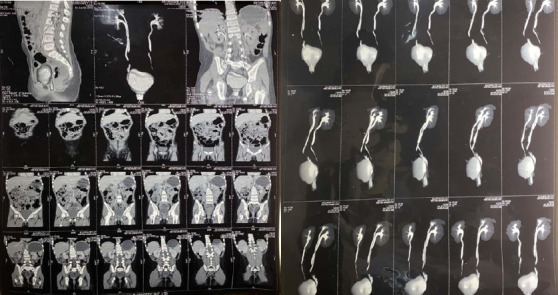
Preoperative CT urogram.
